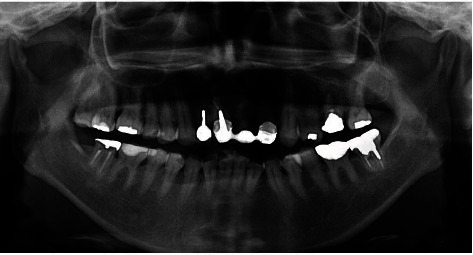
Panoramic radiograph demonstrated the no sign of alveolar bone loss on the transplanted tooth. This patient has maintained all the teeth for almost 30 years from initial appointment.
